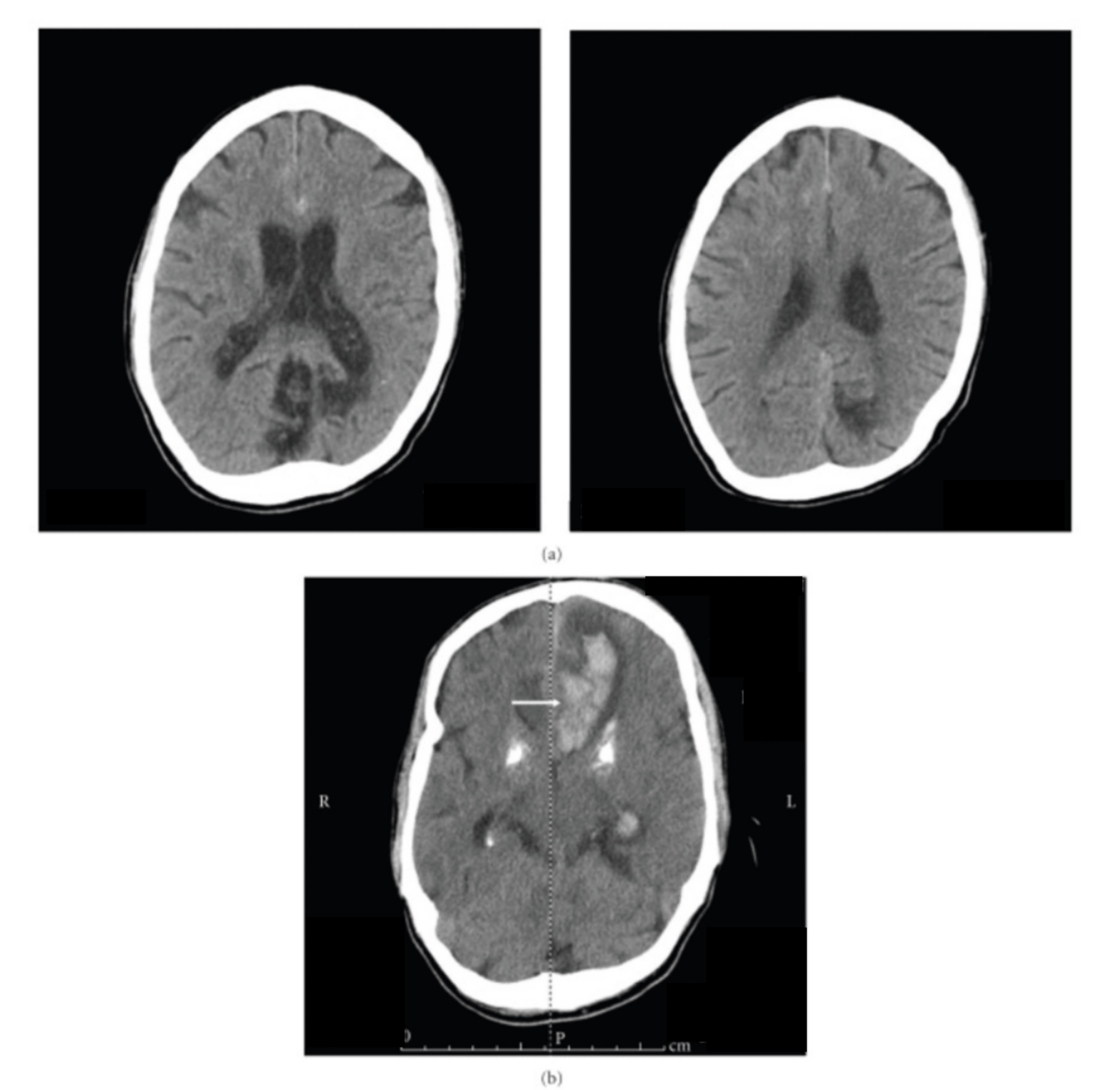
Noncontrast CT brain imaging (a) interhemispheric subarachnoid hemorrhage and (b) intraparenchymal hemorrhage 12 hours later, consistent with ruptured mycotic aneurysm (arrow). Image credit: Kuo et al. [41] (CC BY 4.0)
